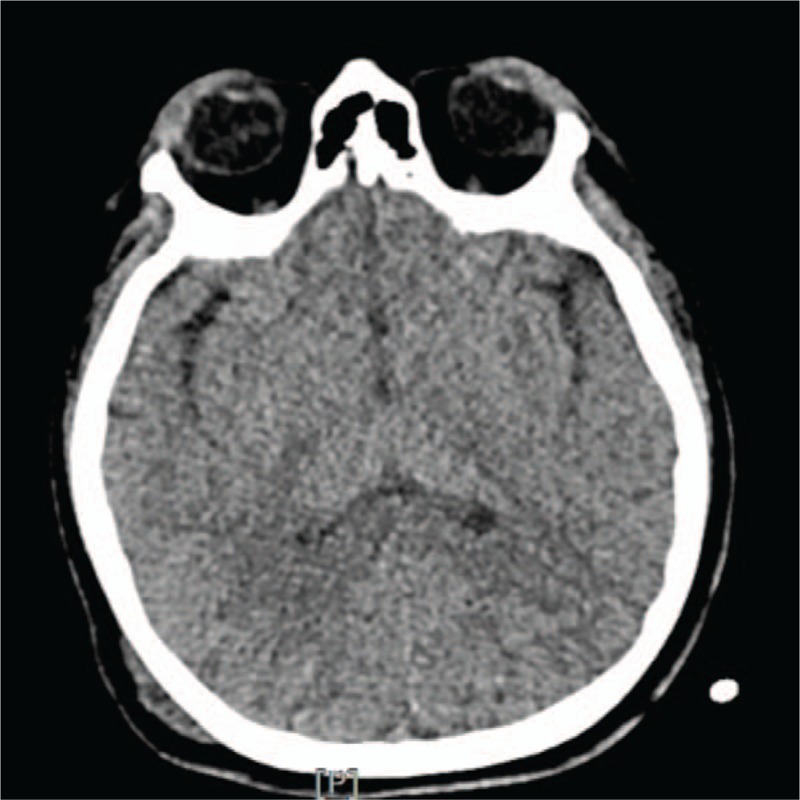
CT of the brain. There are hematoma and emphysema along the right frontal, parietal scalp and both occipital scalp area, but there is no evidence of intracranial hemorrhage. CT = computed tomography (CT).
